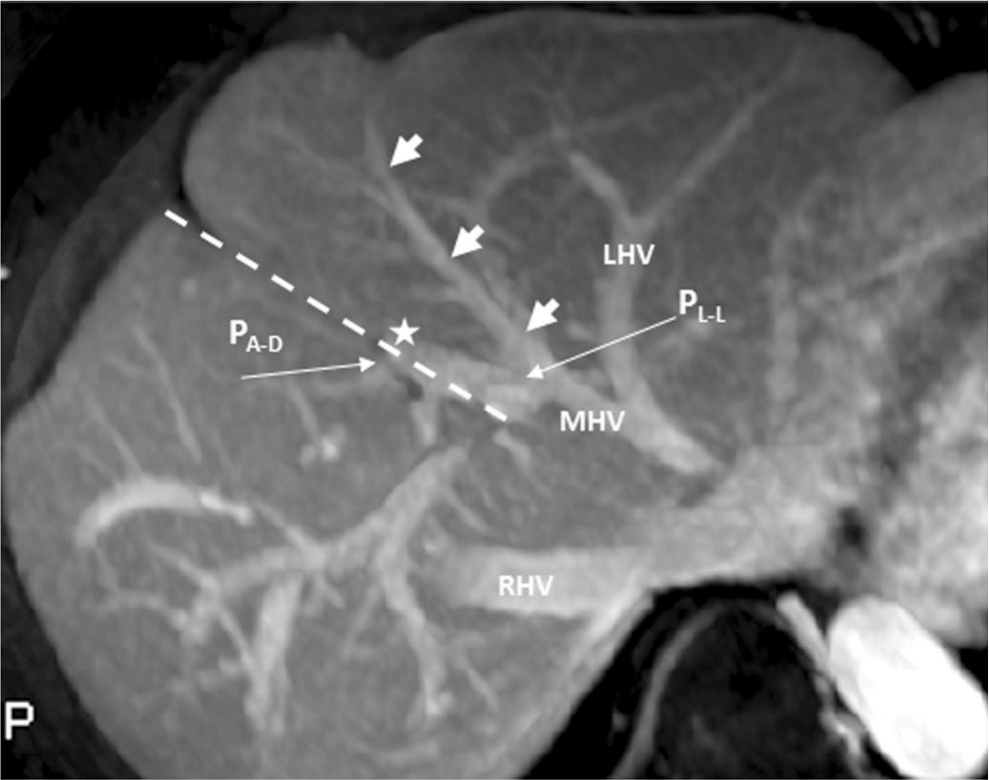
Contrast-enhanced MRI with MIP reconstruction revealing the MHV running to the left (*short arrows*) of the umbilical portion (*star*) and RSLT (*dotted line*), with the umbilical portion (*asterisk*) tilting to the right and joining with the RSLT. The diverging point of the dorsal branch of the right anterior portal vein (P_A–D_) is distal to that of the left lateral portal vein (P_LL_)
